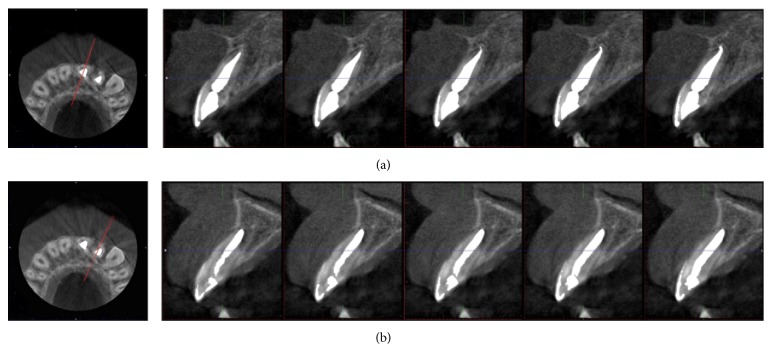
CBCT images of the left upper lateral and central incisor at the 3-year follow-up.
